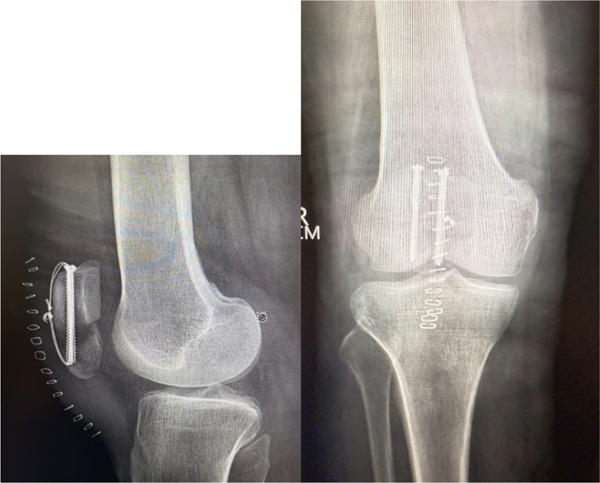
Preoperative lateral and AP radiographs demonstrating hardware failure due to pullout from the inferior pole fragment.
